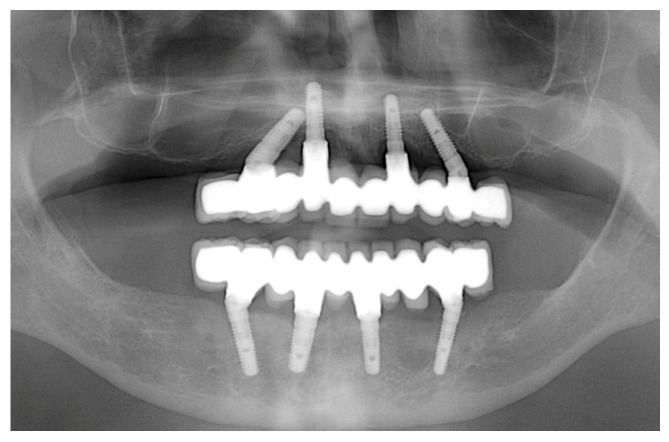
Five-year follow-up orthopantomograph.
